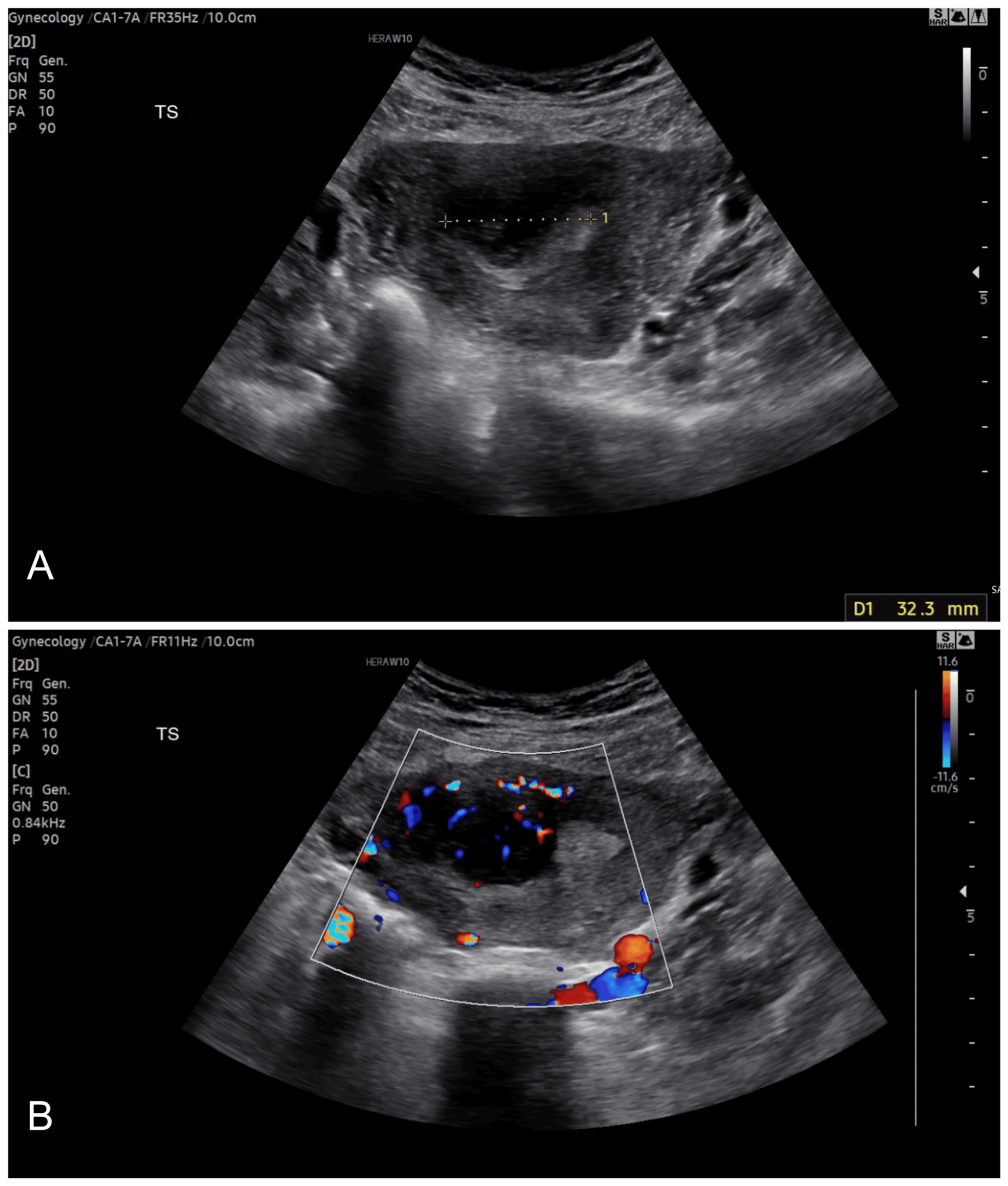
Ultrasound following miscarriage and persistent vaginal bleeding raising suspicion of an abscess showing (A) a uterine heterogeneous mass and (B) high vascularity on Doppler examination
